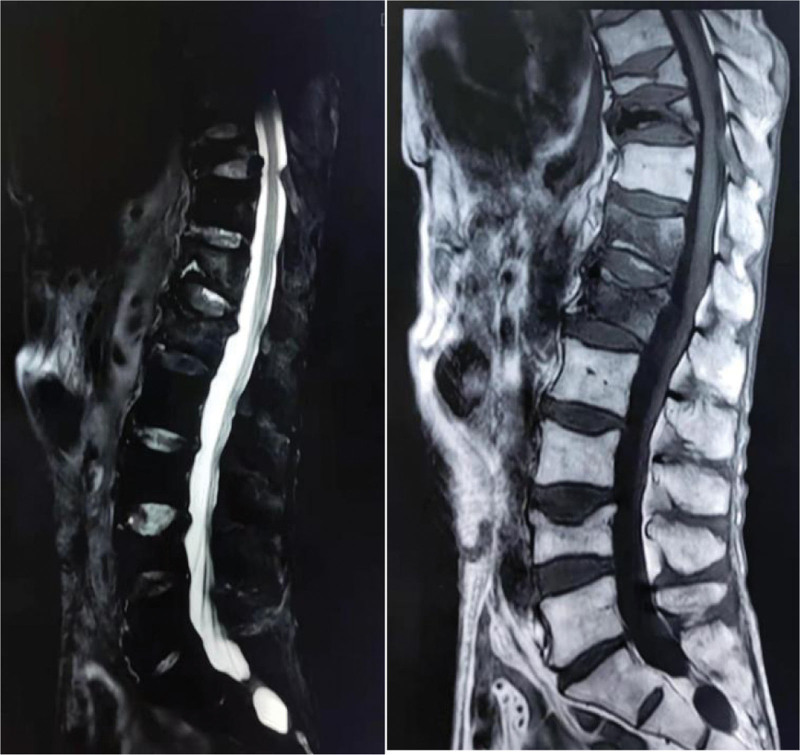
A 85 year old man was treated with PKP for T11 and L 1, but his back pain recurred 1 month after operation. MRI showed the fracture of T12. MRI = magnetic resonance imaging, PKP = percutaneous kyphoplasty.
